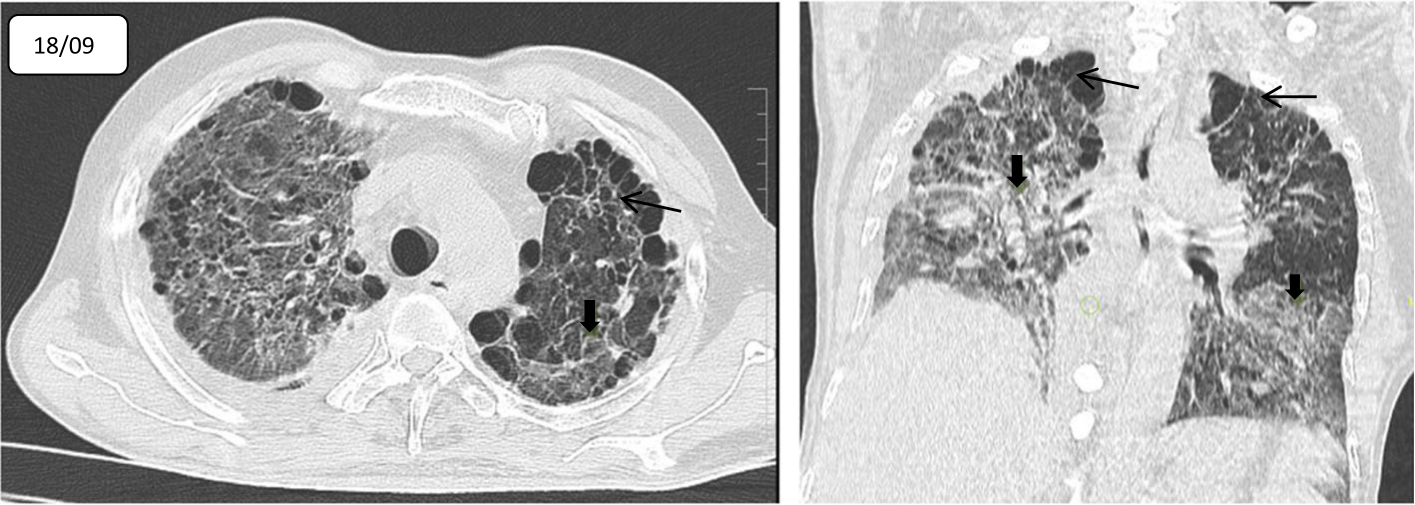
a, b An initial HRCT axial and coronal section shows diffuse ground glass opacities and reticular interstitial thickening (solid black arrow) with emphysematous changes and bulla (line arrow) in bilateral lung fields
